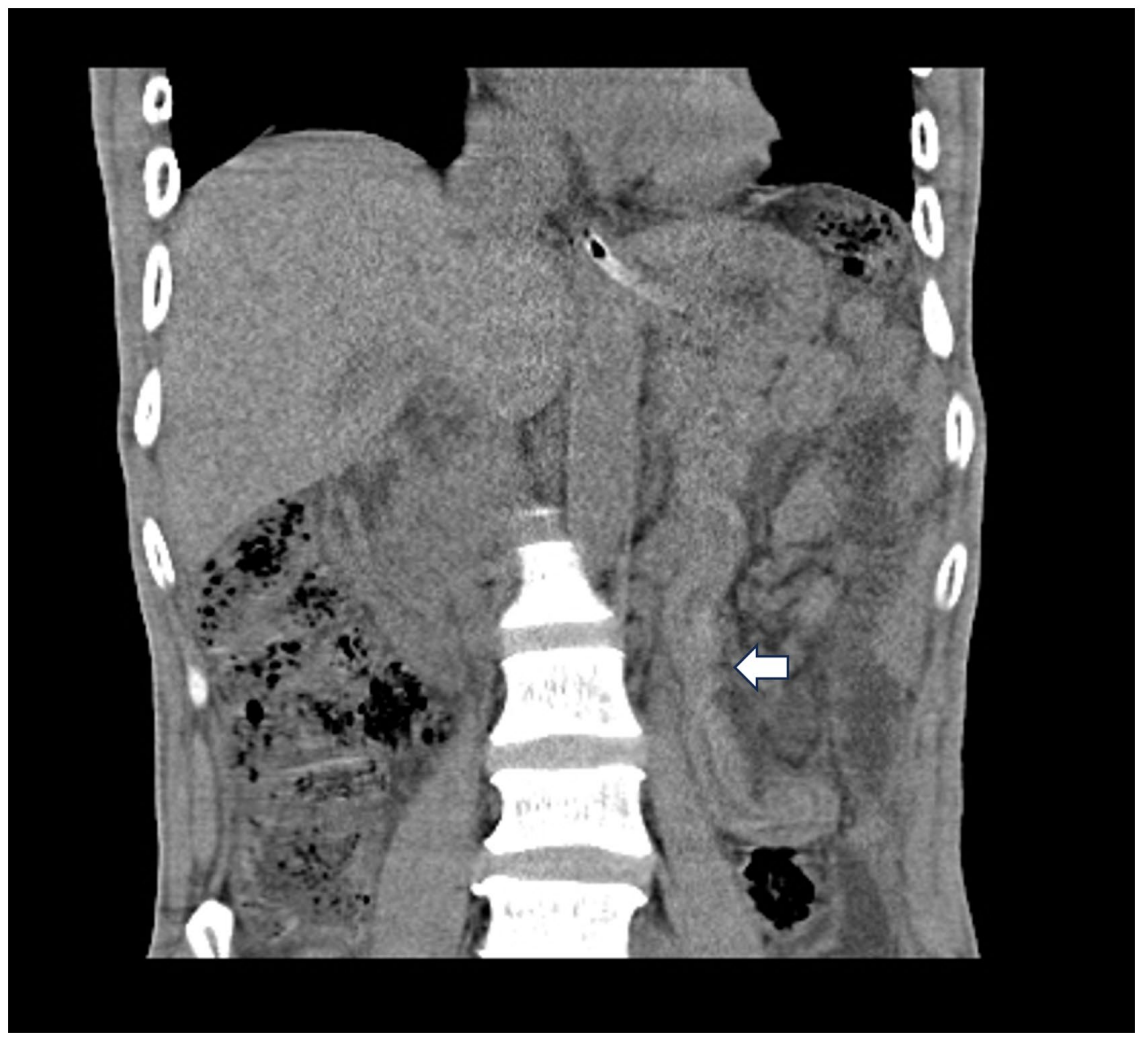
Plain computed tomography of the abdomen revealed a stringy shadow in the jejunum (white arrow).
